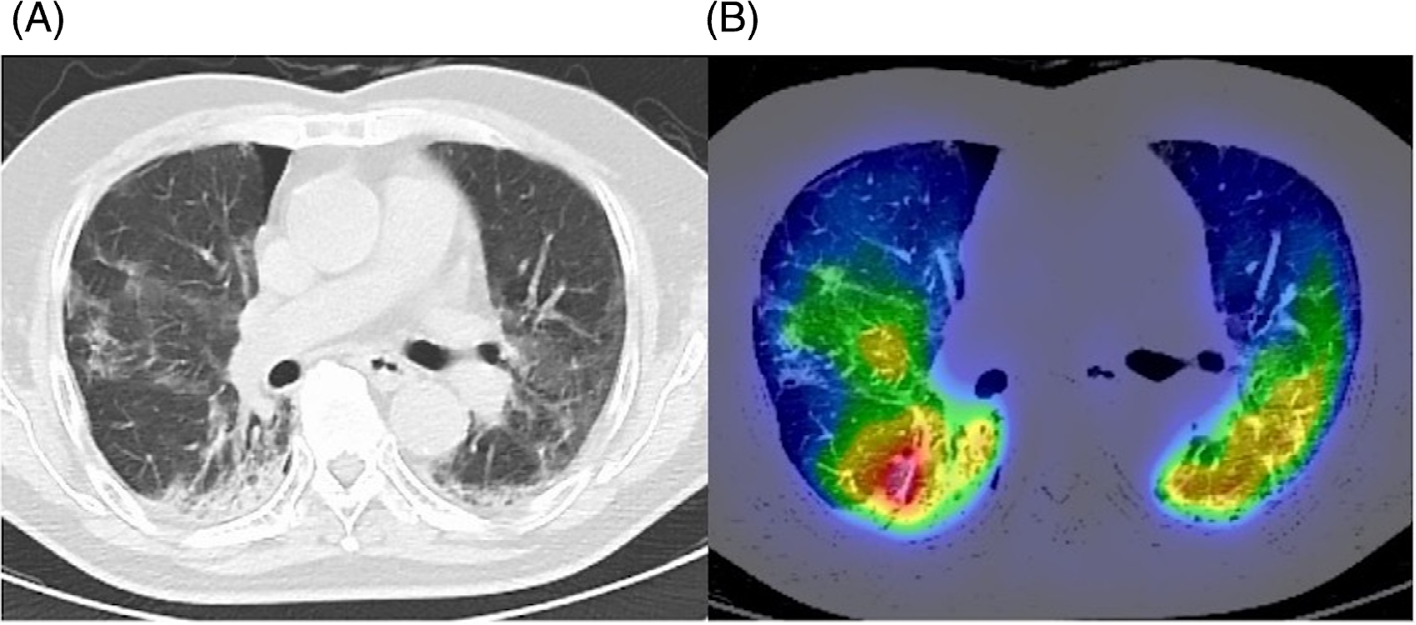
(A, B) A ventilation/perfusion single‐photon emission computed tomography combined with computed tomography (V/Q SPECT/CT) showed perfusion uptake within and around the fibrotic lesions in the lower lobe bilaterally. CT, computed tomography
